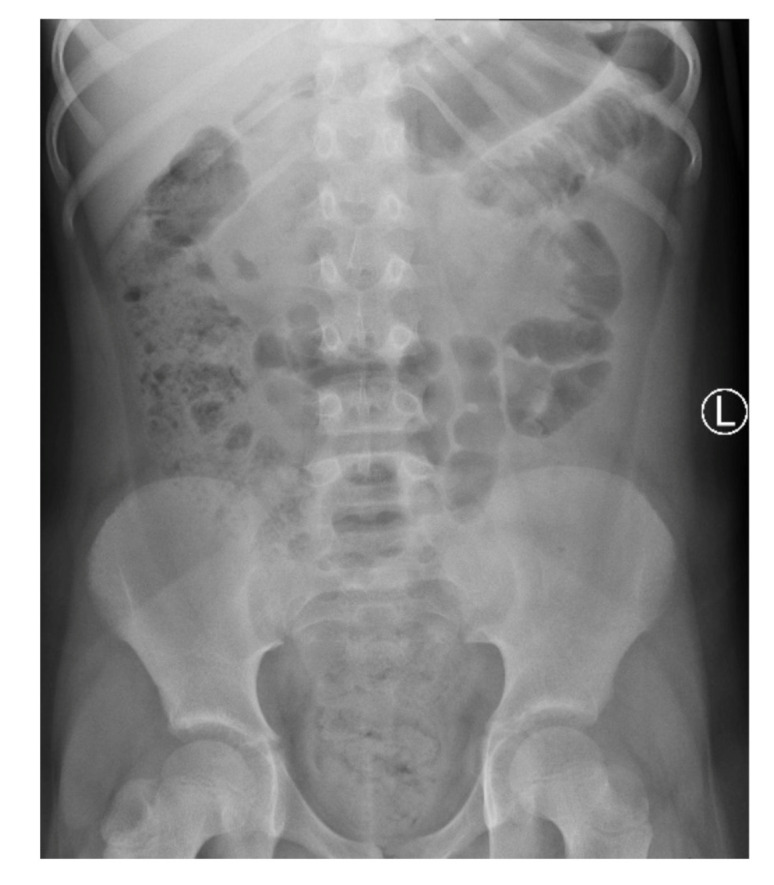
A plain X-ray of the abdominal cavity on admission: no signs of obstruction or perforation of the gastrointestinal tract and fecal masses in the large intestine.
